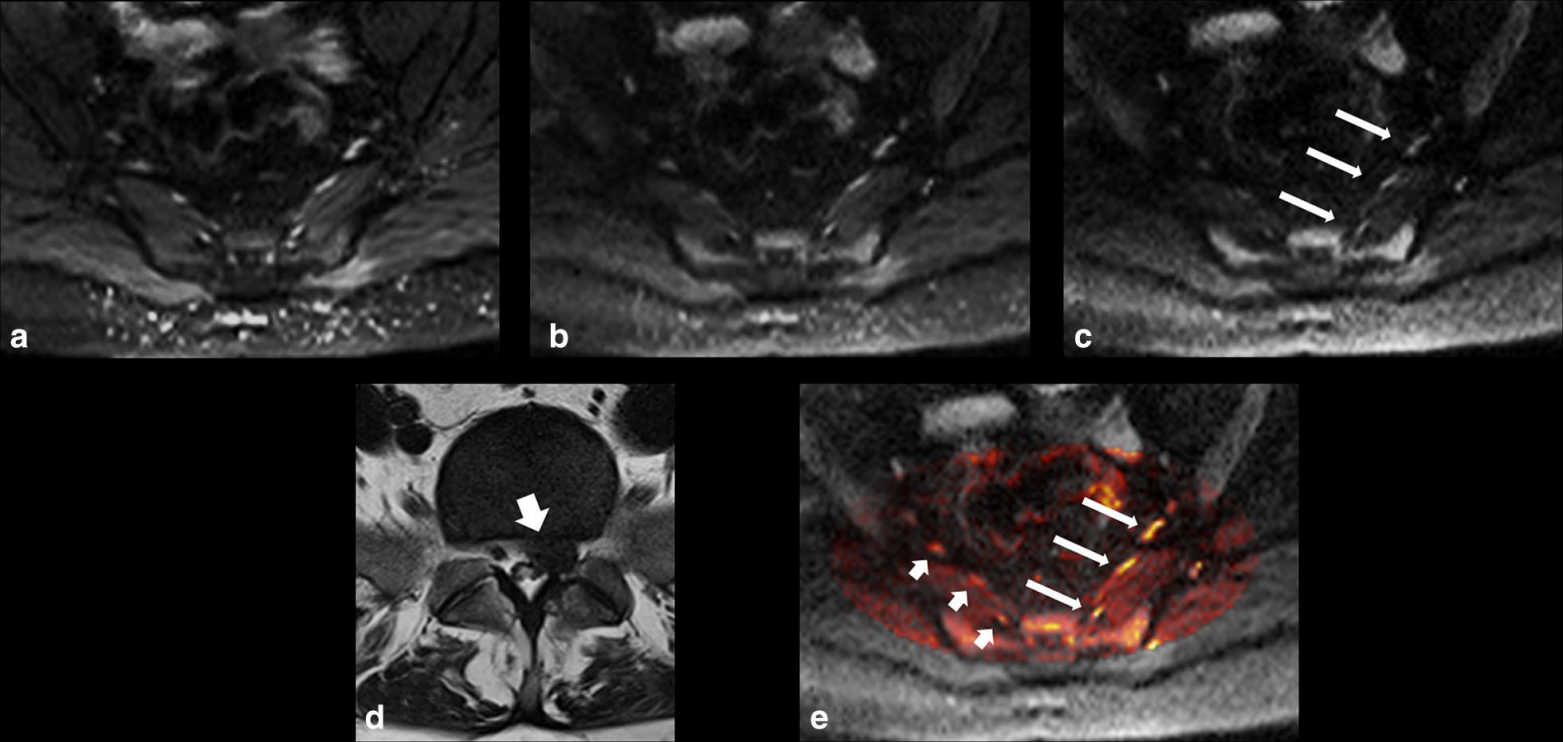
45-year-old female suffering from left leg pain, severe weakness and numbness. DWI-RESOLVE images (a) *b* = 50, (b) *b* = 500, (c) *b* = 800. The arrows on the b800 image correlate to the left L5, S1 and S2 nerves from anterior to posterior. The nerves were evaluated by both readers on axial DWI-RESOLVE images as positive for abnormality (grade III abnormal size and signal intensity). Axial T2W TSE d of the lumbar spine at L5/S1 highlights a large left-sided disc herniation (arrow), which explains symptoms and correlates to nerve abnormalities. The color-mapped image e demonstrates the asymmetry of the lumbosacral plexus to better effect in this patient. Long arrows indicate abnormal left nerves and short arrows indicate normal contralateral side
